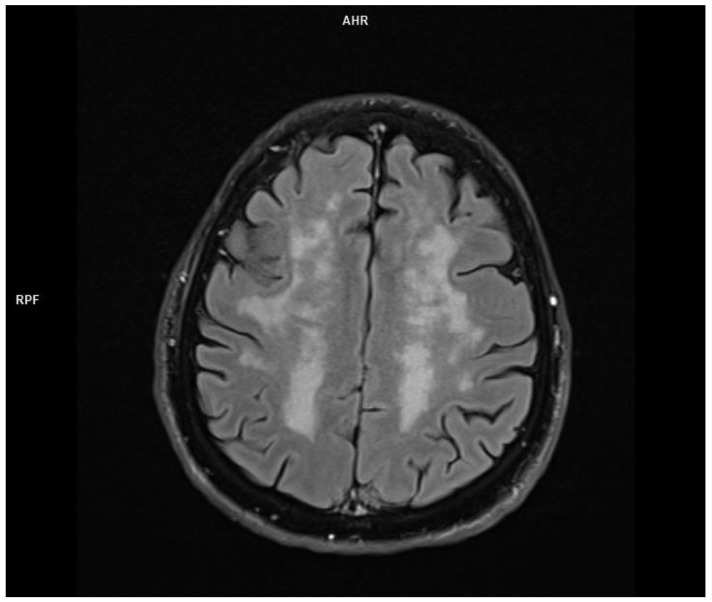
Diffuse hyperintense areas of the subcortical white matter—Flair sequence (MRI imaging).
